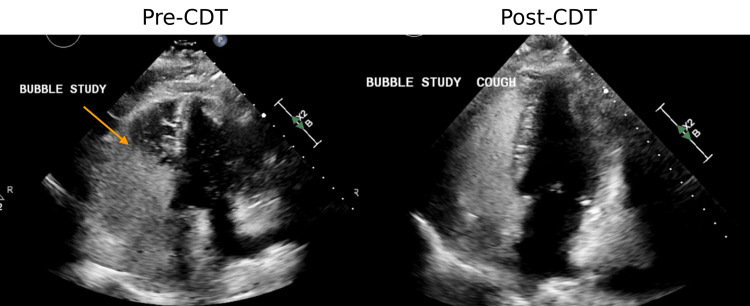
Pre-CDT (left), echocardiogram images demonstrated a positive bubble study indicative of a PFO. Following treatment of the acute PE with CDT (right), the bubble study was negative, suggestive of PFO closure. CDT: catheter-directed thrombolysis, PFO: patent foramen ovale, PE: pulmonary embolism.
